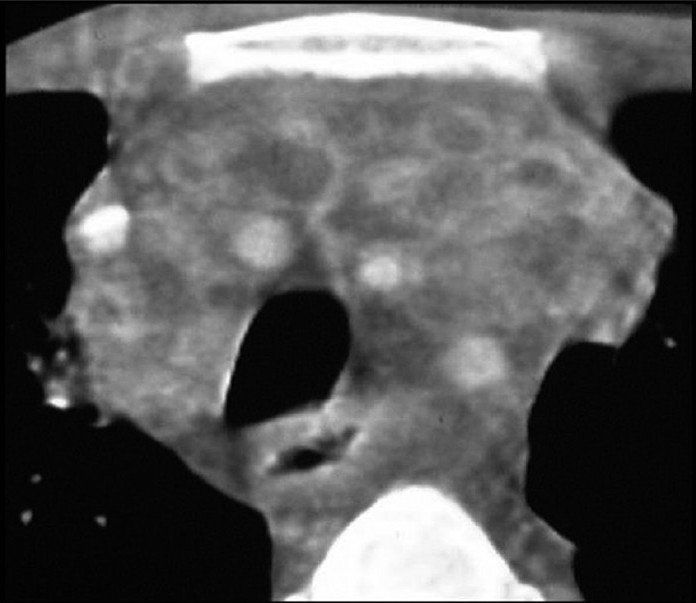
Mycobacterium tuberculosis* (TB). TB mediastinal lymph nodes are typically markedly enlarged and of low attenuation on computed tomography, often demonstrating rim enhancement following contrast administration, as in this case
